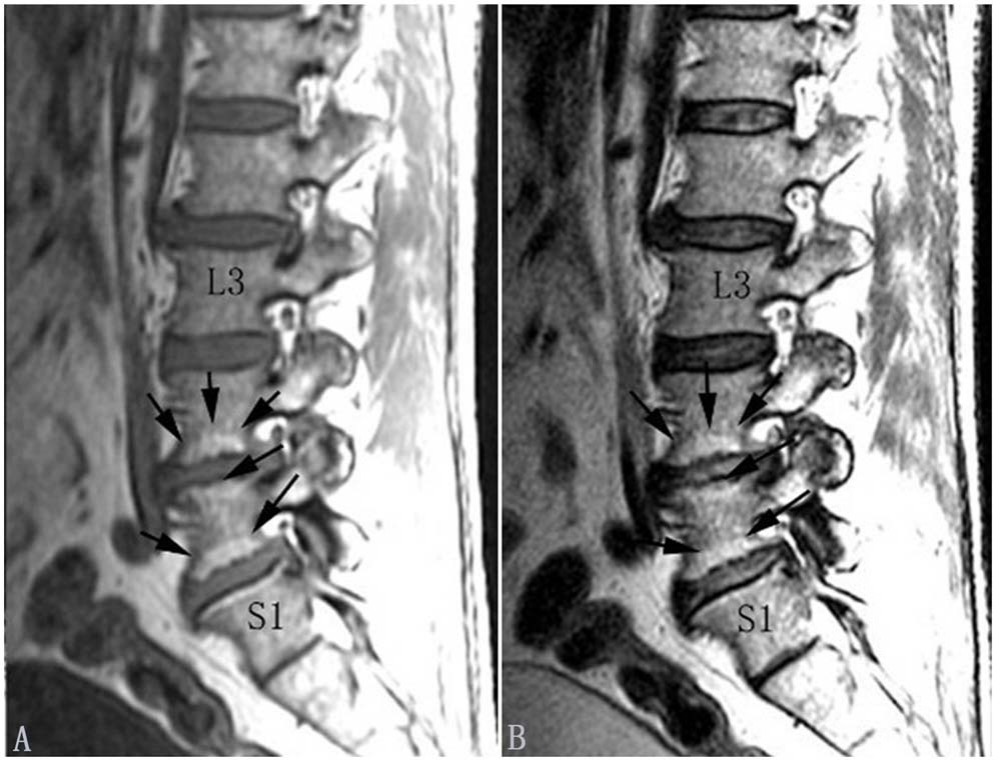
Modic type II changes in the lumbar spine (indicated by arrows). Modic type II changes appear hyperintense on T1-weighted (A) and isointense or hyperintense on T2-weighted images (B).
